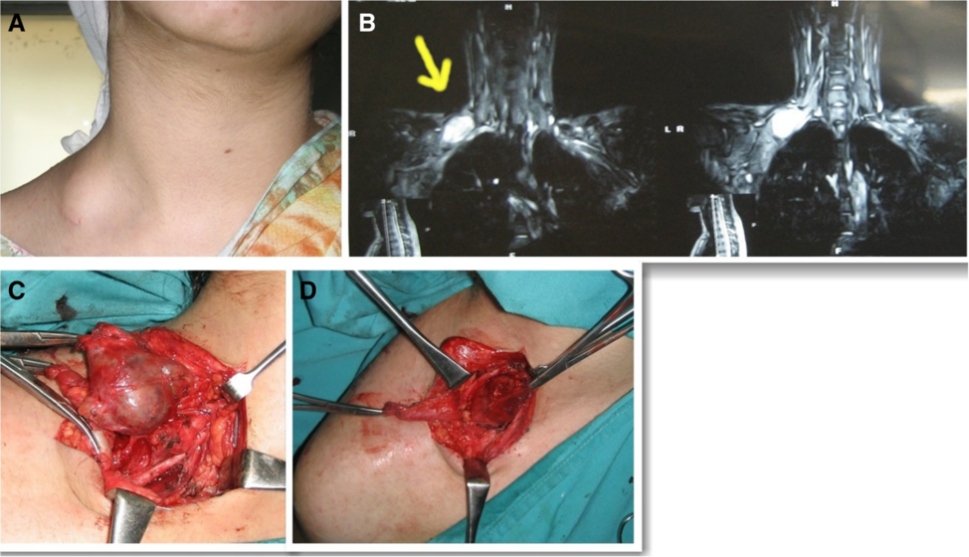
(A) Shwannoma right Supraclavicular region, (B) MRI showing T2 weighted image enhancement, (C) peroperative enucleation, (D) After enucleation.
